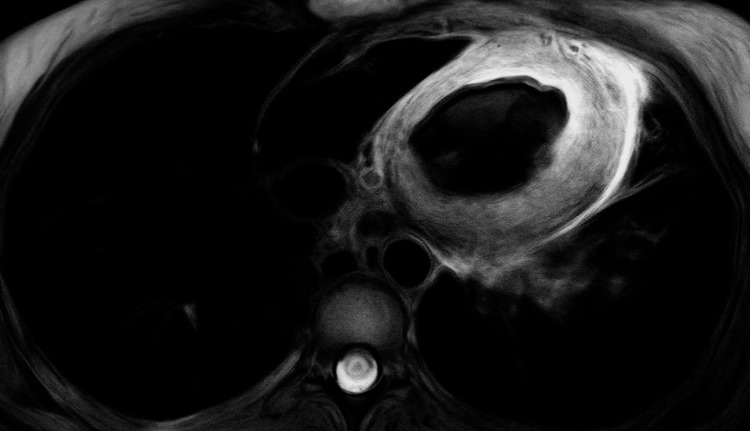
CMR with short-axis view showed extensive myocardial edema on T2-weighted fat-suppressed imaging CMR: Cardiac magnetic resonance imaging
